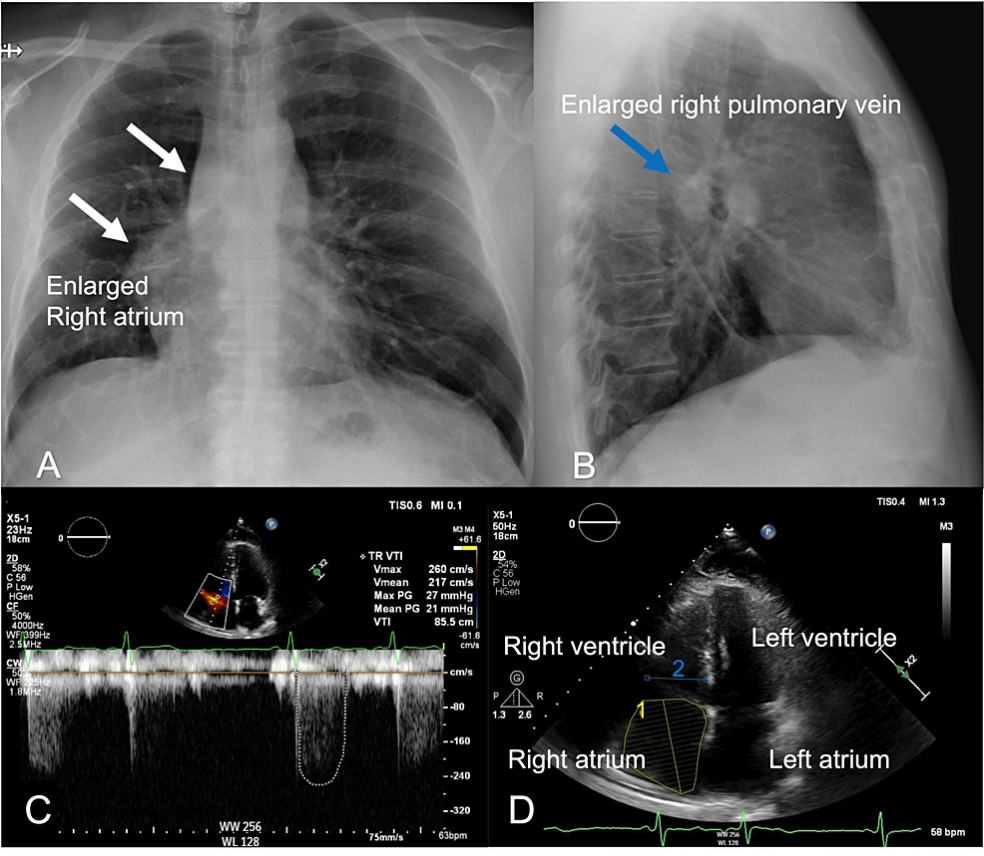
(A) Posteroanterior and (B) lateral chest radiograph demonstrating an enlarged right heart border (white arrows) and enlarged right pulmonary vein (blue arrow). Transthoracic echocardiogram with four-chamber apical views revealing (C) mild tricuspid regurgitation and (D) enlargement of right heart chambers.
